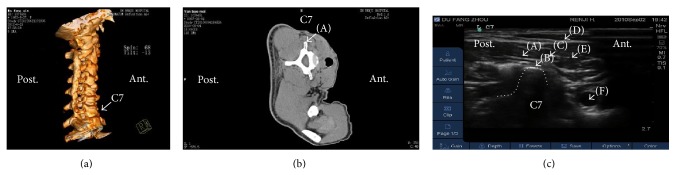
Ultrasound identification of the seventh cervical vertebra (C7) during the UG-MSN and UG-DN techniques. (a) Three-dimensional computed tomography reconstruction of the cervical vertebrae. The white arrow indicates C7. (b) Conventional computed tomography scanning of C7. (A) The posterior tubercle of the transverse process of C7. (c) Ultrasound imaging of C7. The dotted line represents the posterior tubercle of the transverse process of C7. The letters (A)–(F) indicate, respectively, the medial scalenus muscle, posterior tubercle, brachial plexus, sternocleidomastoideus muscle, anterior scalenus muscle, and cervical artery. Post.: posterior position of patients. Ant.: anterior position of patients.
